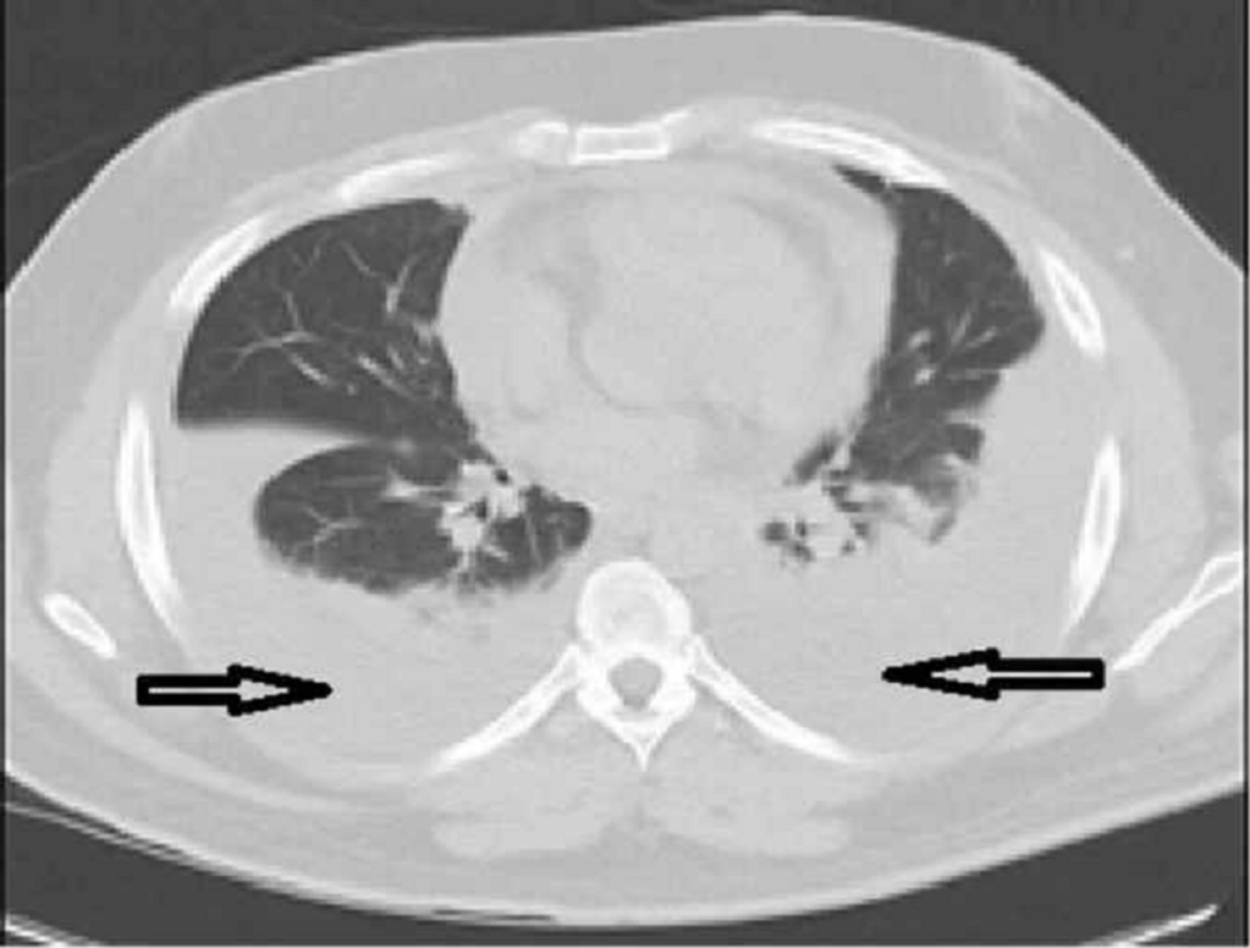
Computed tomography of chest (lung window) showing pericardial effusion and bilateral pleural effusion Arrows pointing pleural and pericardial effusion
